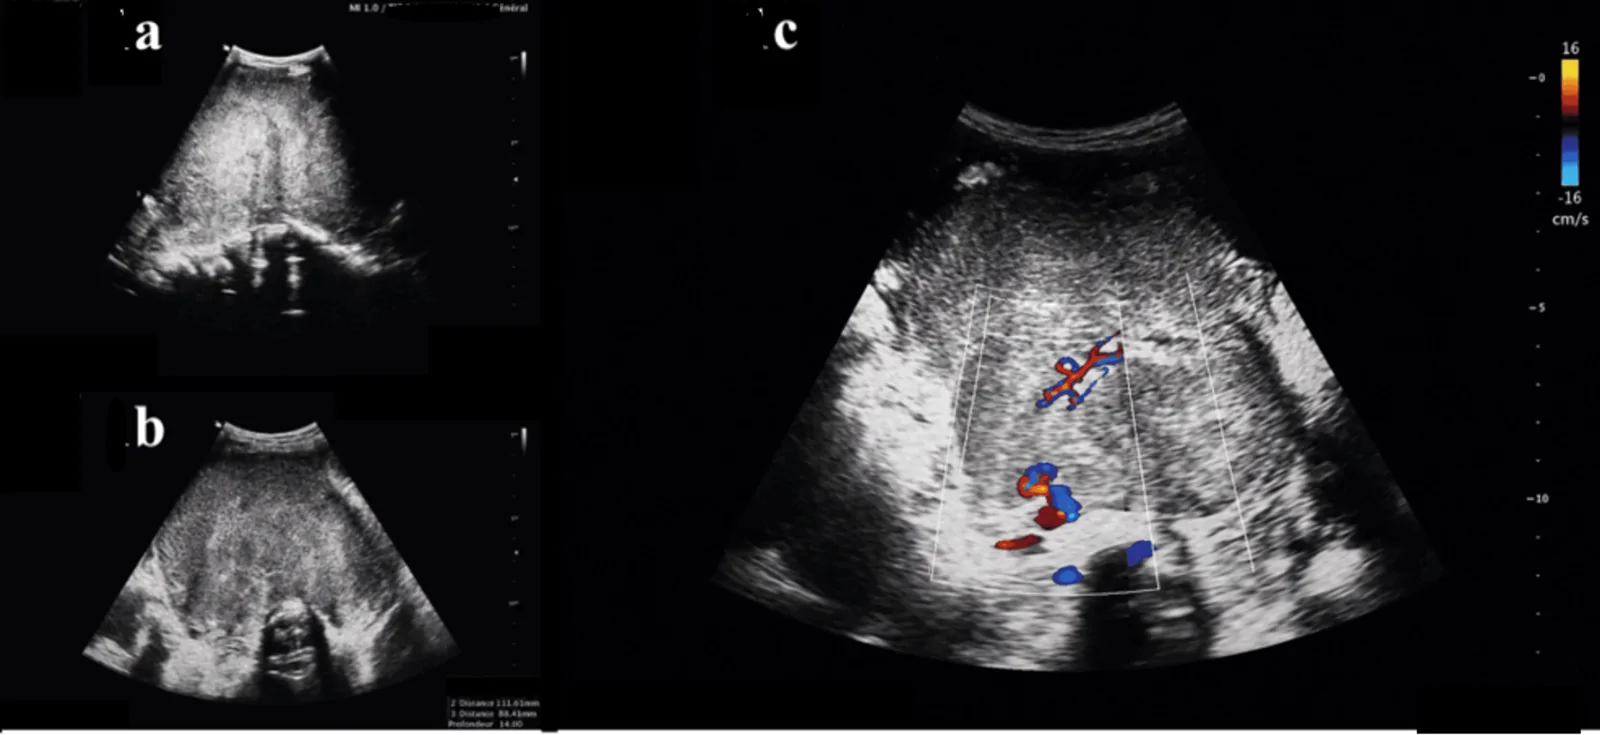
Initial abdominal ultrasound. (a and b) Grayscale image showing a large, well-defined, heterogeneous prevertebral retroperitoneal mass. (c) Color Doppler image showing sparse internal vascularity.
